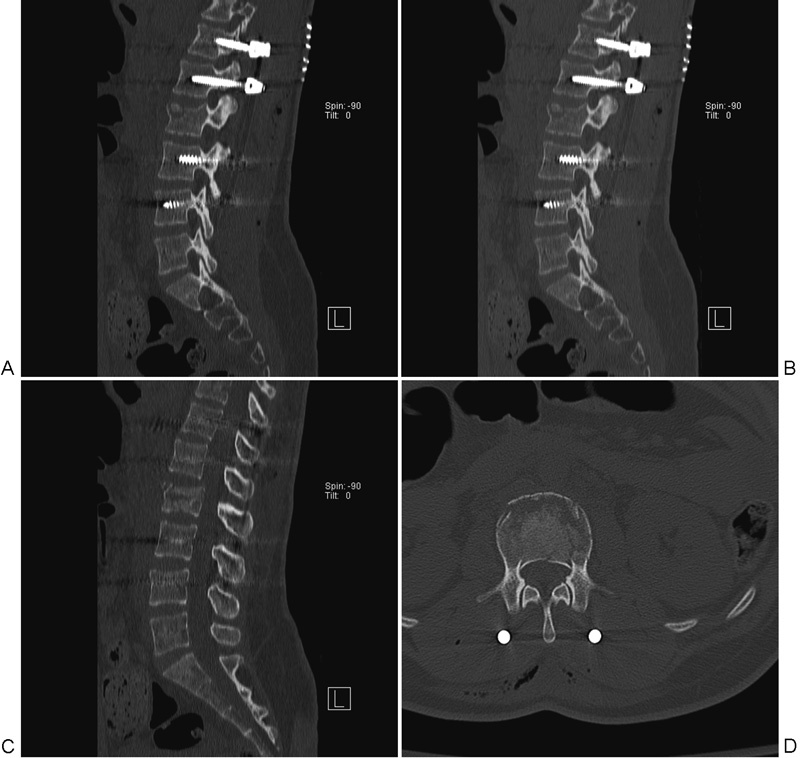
Postoperative computed tomography assessment of lumbar spine. Significant improvement of L2 vertebra loss of height, Cobb angle, and canal compromise is noted. (A) Sagittal view of left-side screw placement. (B) Sagittal view of right-side screw placement. (C) Midline sagittal view. (D) Axial view.
